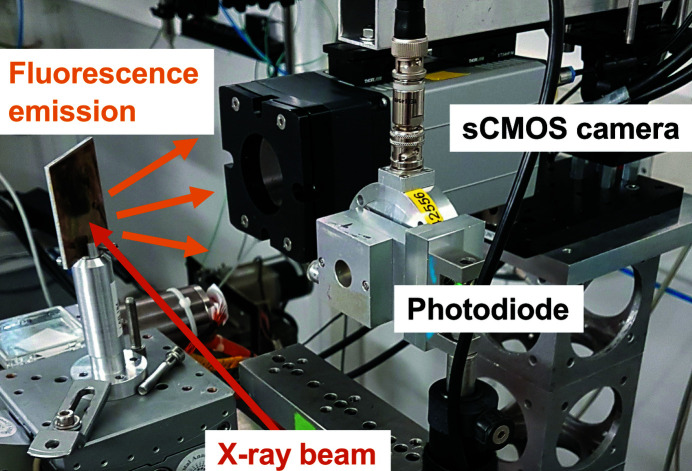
The experimental setup for measurement of single X-ray photons. The fluorescent target is oriented at 45° and the camera at 90°, with respect to the beam path.
